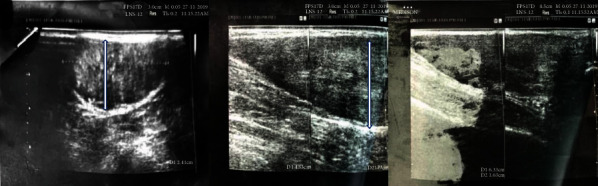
Ultrasound appearance of thickened Achilles tendon with focal hypoechoic areas. White arrows, thickened Achilles tendon.
